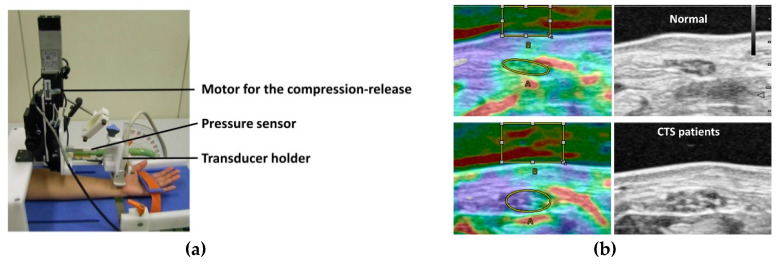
Pressure-monitor ultrasound system for the quantitative assessment of median nerve strain. (a) Pressure-monitor ultrasound system, (b) elastographic images of CTS patients and normal controls. Upper row shows the image of a normal subject. Lower row shows the image of a CTS patient.
